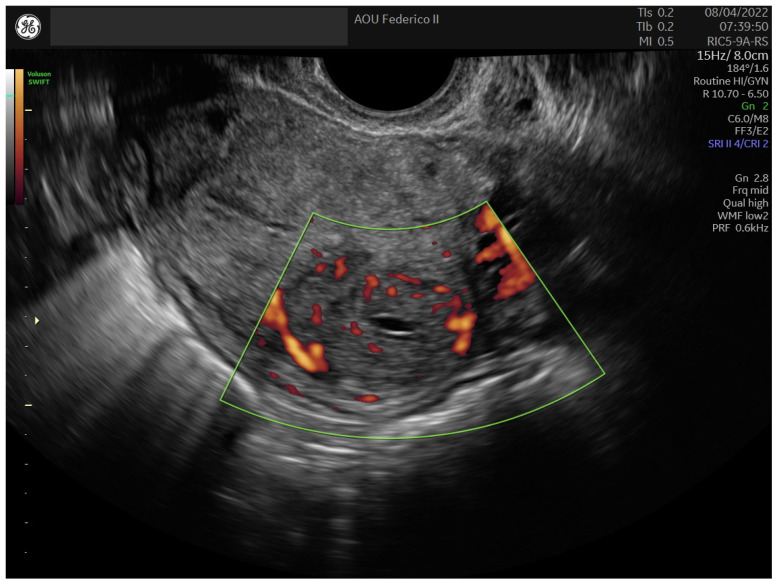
Sonographic Power-Doppler appearance of the UTROSCT in Case 1.
